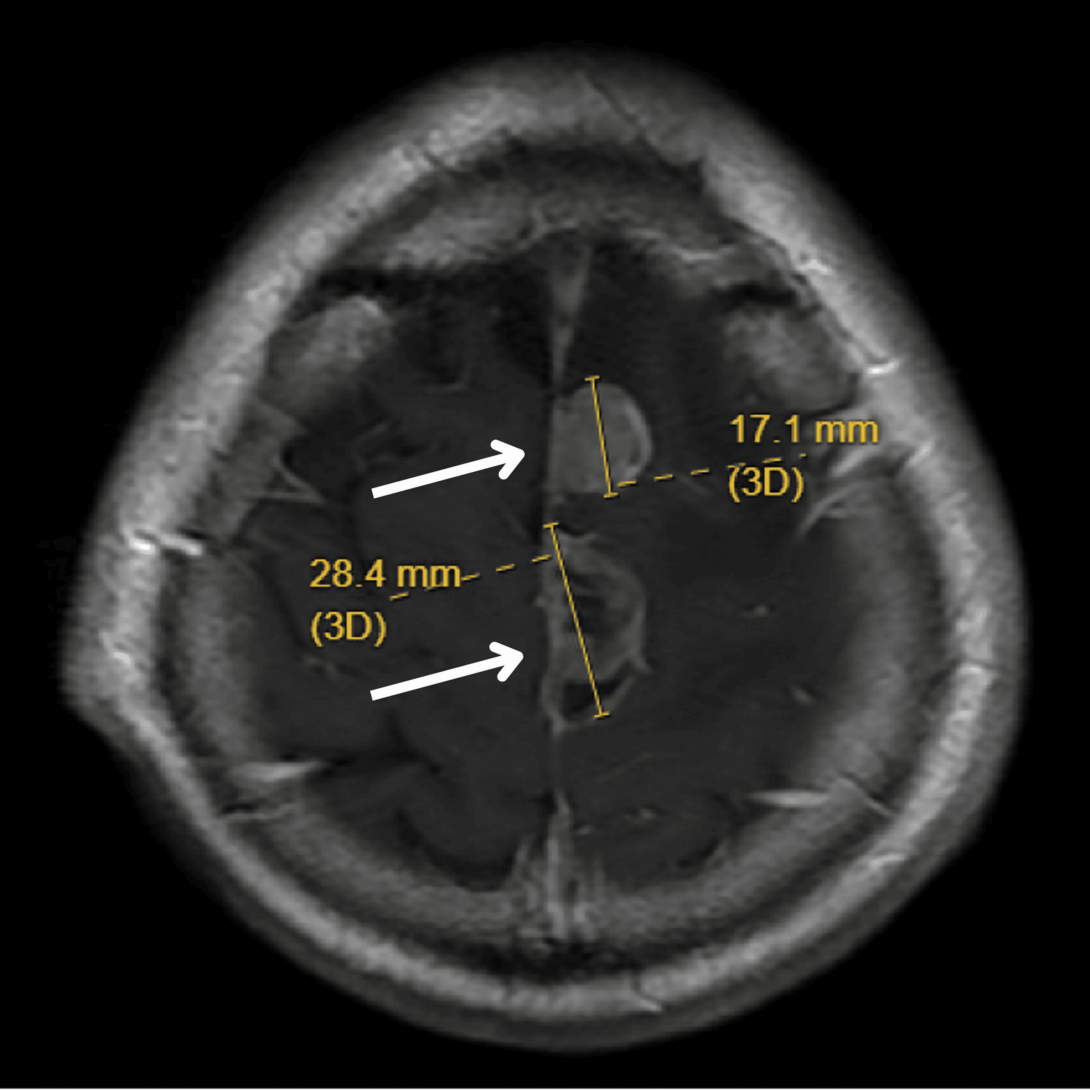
Disease progression on surgical resection and radiotherapy. Axial T1-weighted contrast-enhanced MRI brain image demonstrating new parasagittal dural metastasis, occurring three months after surgical resection and stereotactic radiotherapy. The patient developed status epilepticus at this time and was treated with palliative whole-brain radiotherapy.
